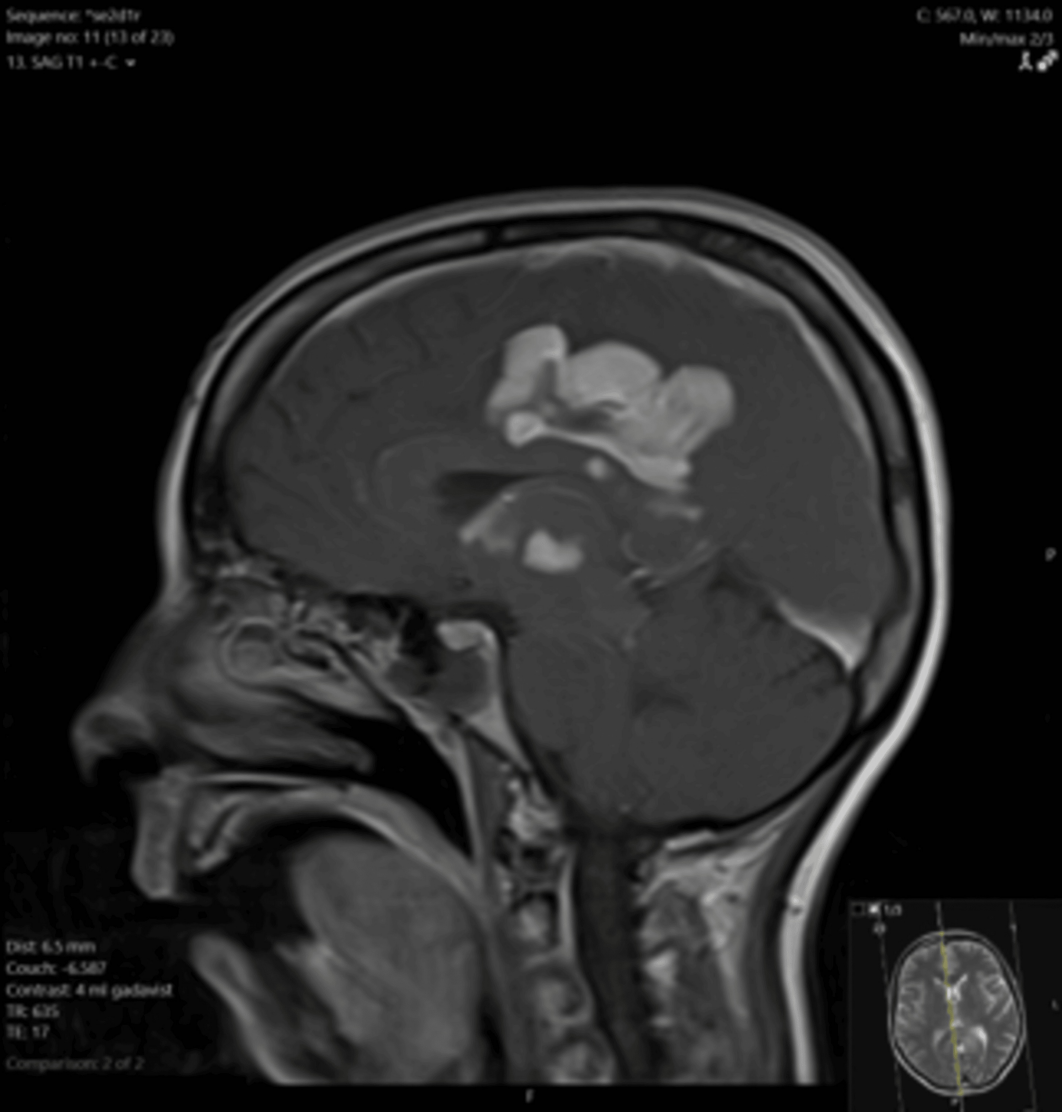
T1 post-contrast sagittal MRI image with avidly enhancing lesions in the right cingulate gyrus, corpus callosum, basal ganglia, and hypothalamus MRI: magnetic resonance imaging
